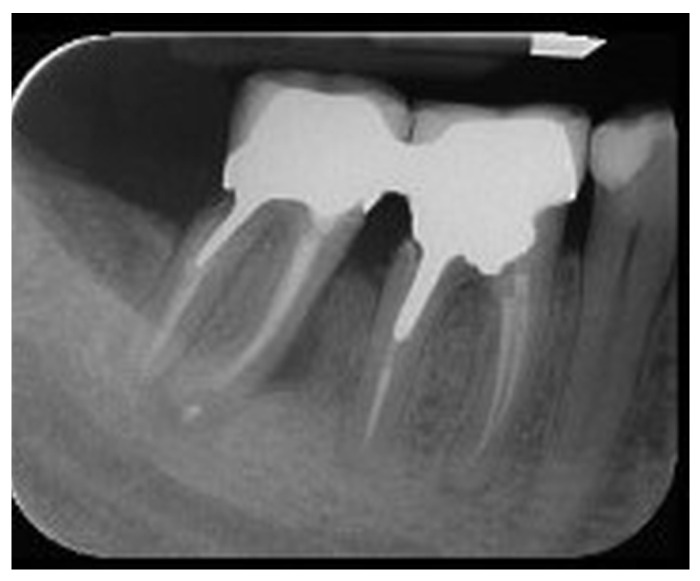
Periapical X-ray of #46, #47.
